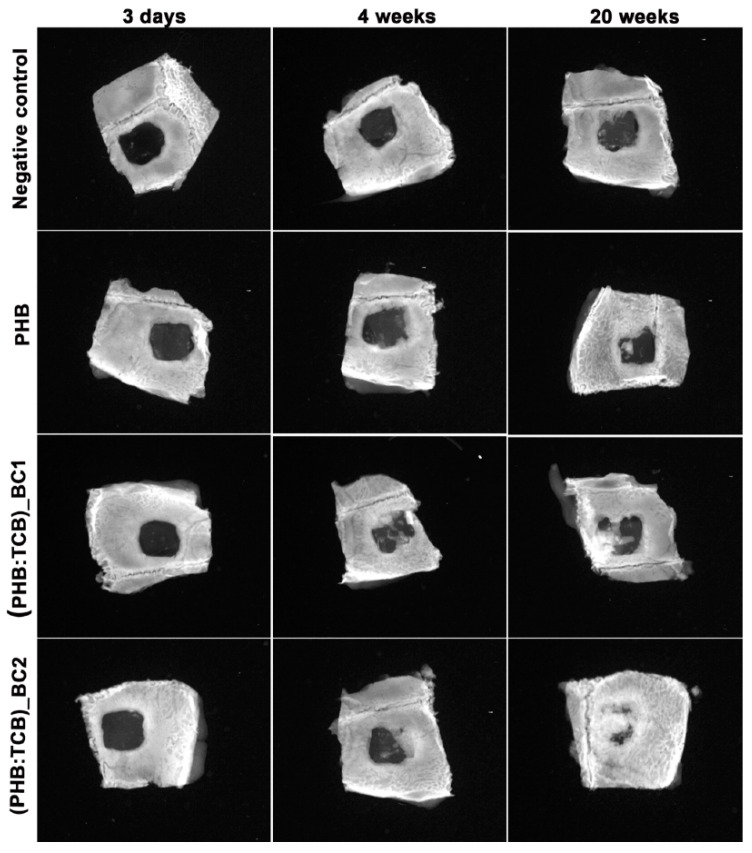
Radiographs of in vivo bone samples treated with scaffolds at different days (3 days, 4 weeks, and 20 weeks). Reprinted with permission [92].
